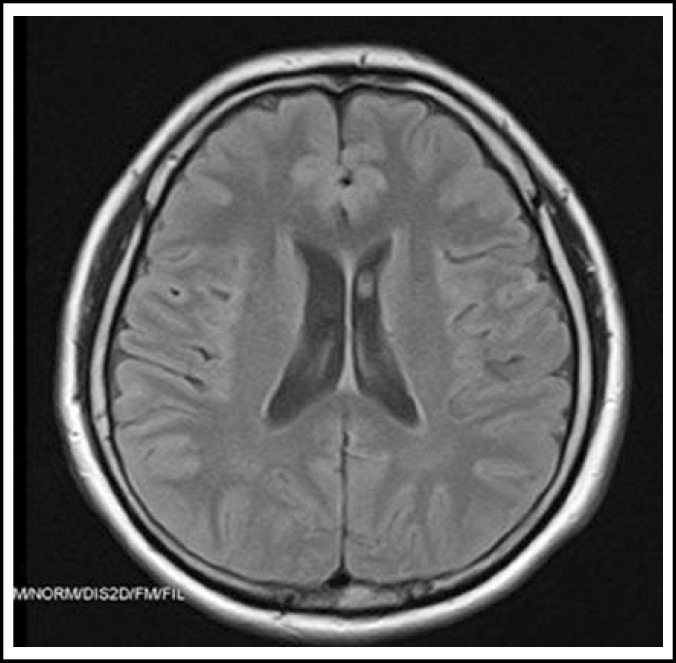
MRI one week following treatment clearly showing marked reduction of cortical enhancement
